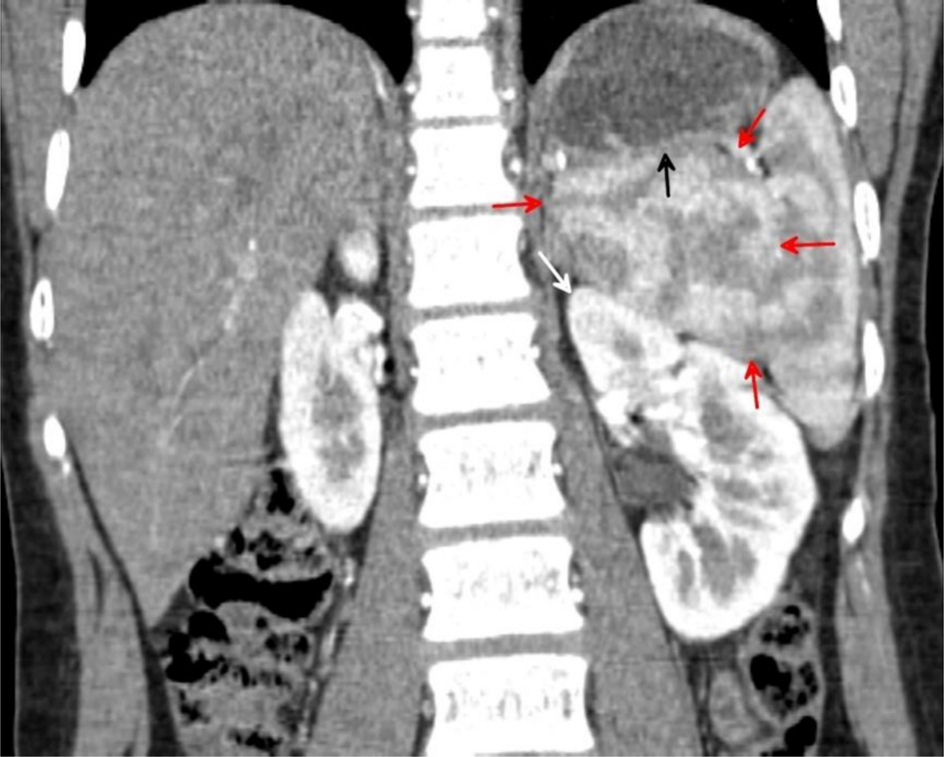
Coronal multiplanar reformation CT image acquired during the arterial phase demonstrates the medial (internal) lobe of the spleen (red arrows), extending medially displacing the upper pole of the left kidney (white arrow) downwards and gently indenting posterior aspect of the gastric fundus (black arrow). The upper pole of the right kidney seen higher than its left counterpart. The medial splenic lobe shows the characteristic mottled appearance of splenic parenchyma on arterial phase.
